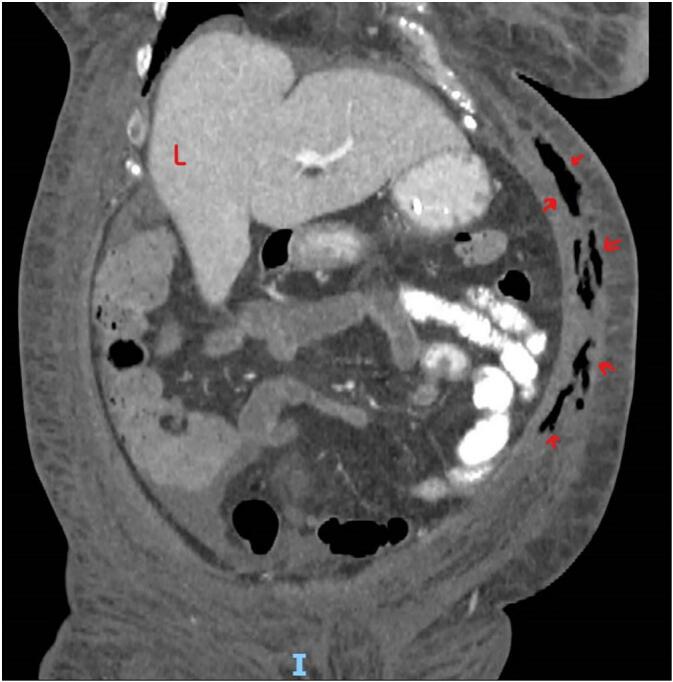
Coronal CT abdomen with air in the abdominal wall (arrows); L liver.
